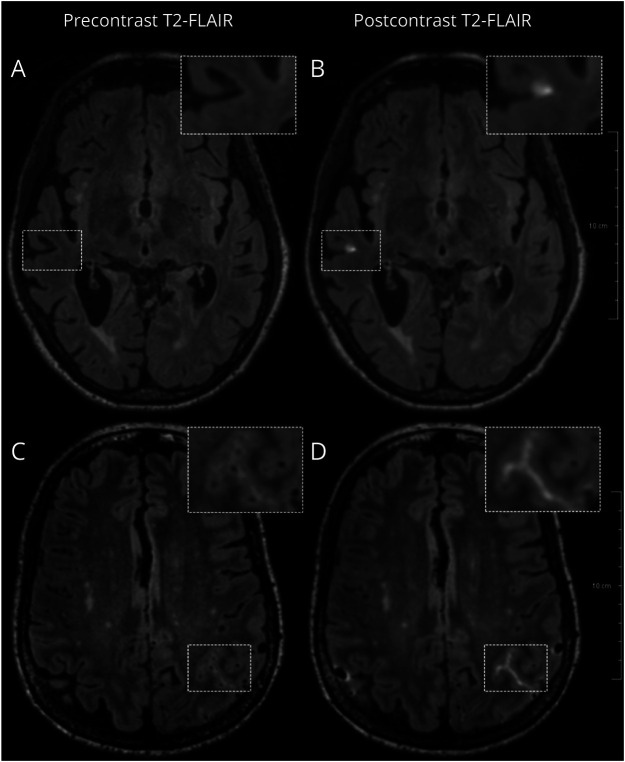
An Example of Focal (A and B) and Sulcal (C and D) CSF Enhancement in 2 Participants With Probable CAA CAA = cerebral amyloid angiopathy; FLAIR = fluid-attenuated inversion recovery.
